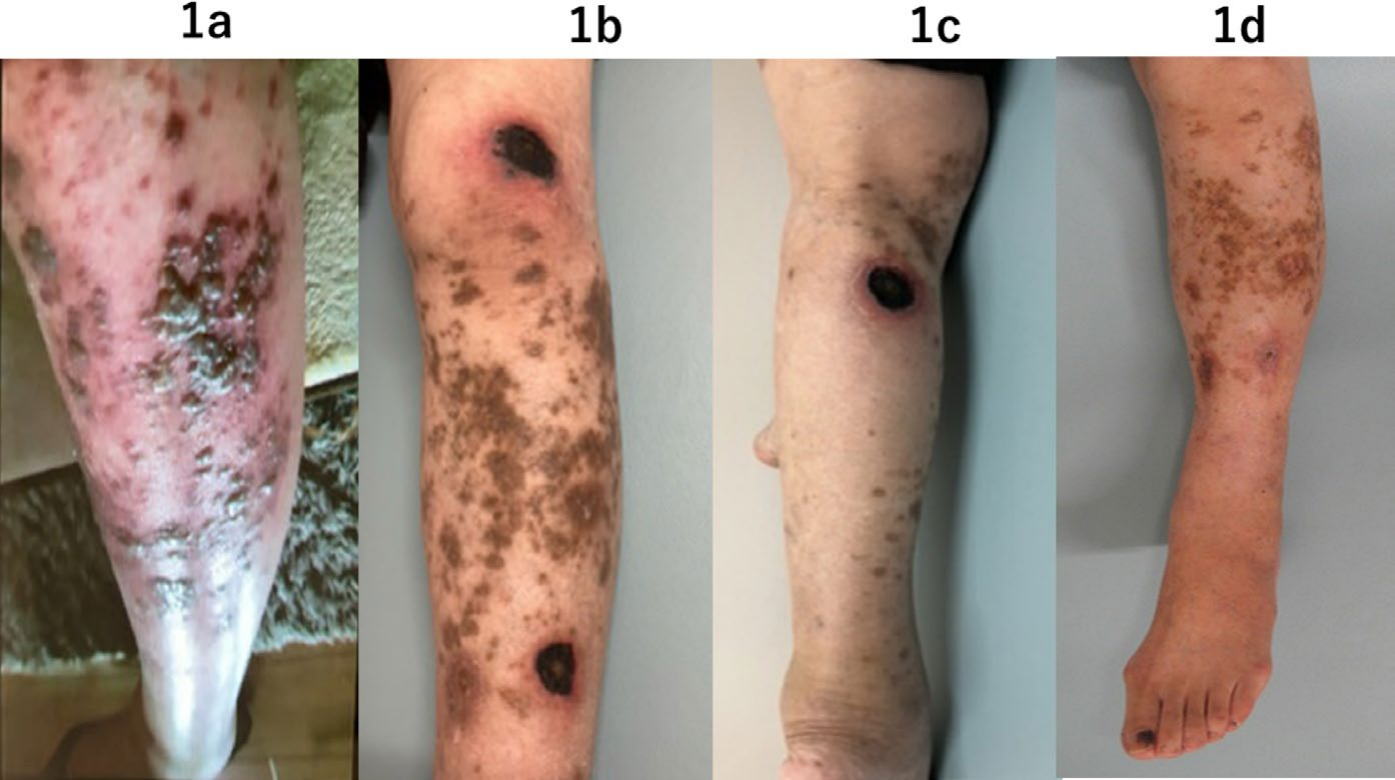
(a) This image shows the left lower limb at the onset of herpes zoster. Multiple blisters and blood blisters have formed on the outer side of the limb, accompanied by redness and swelling. (b) This is the initial finding at our hospital, 4 months after the onset of herpes zoster. The left lower limb exhibits swelling, band‐like pigmentation, and ulcers with necrosis and crusting in a circular pattern. (c) In the left popliteal fossa, a large necrotic crust is visible. Ultrasound revealed a massive thrombus just beneath this area. (d) This image shows the left lower limb after complete epithelialization. The edema has subsided, but significant hyperpigmentation remains.
